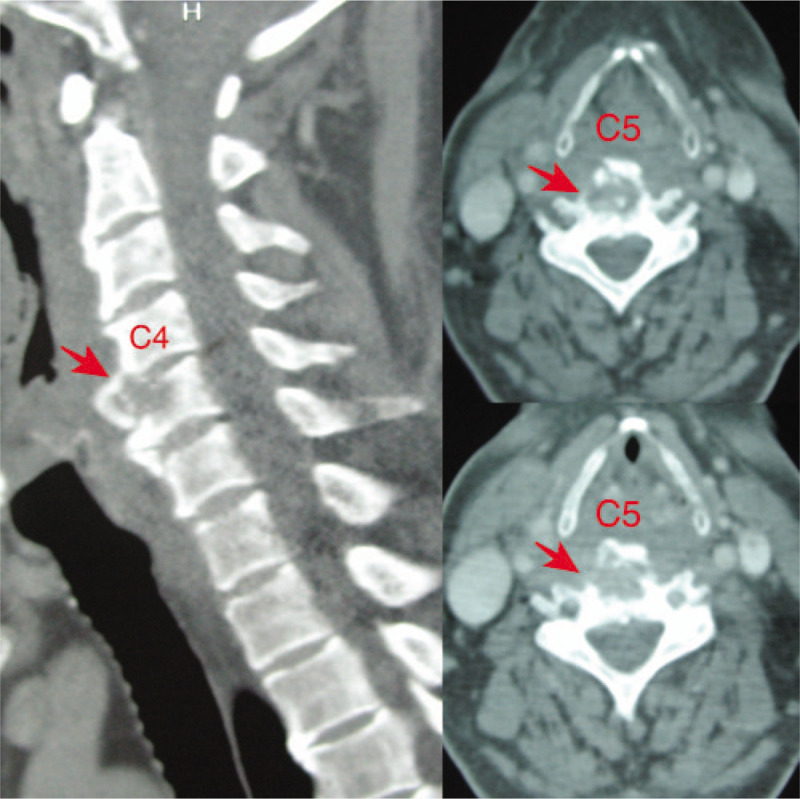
CT scan of the cervical spine showed that the C4 and C5 vertebral bodies were eroded and destroyed (arrow). CT = computed tomography.
